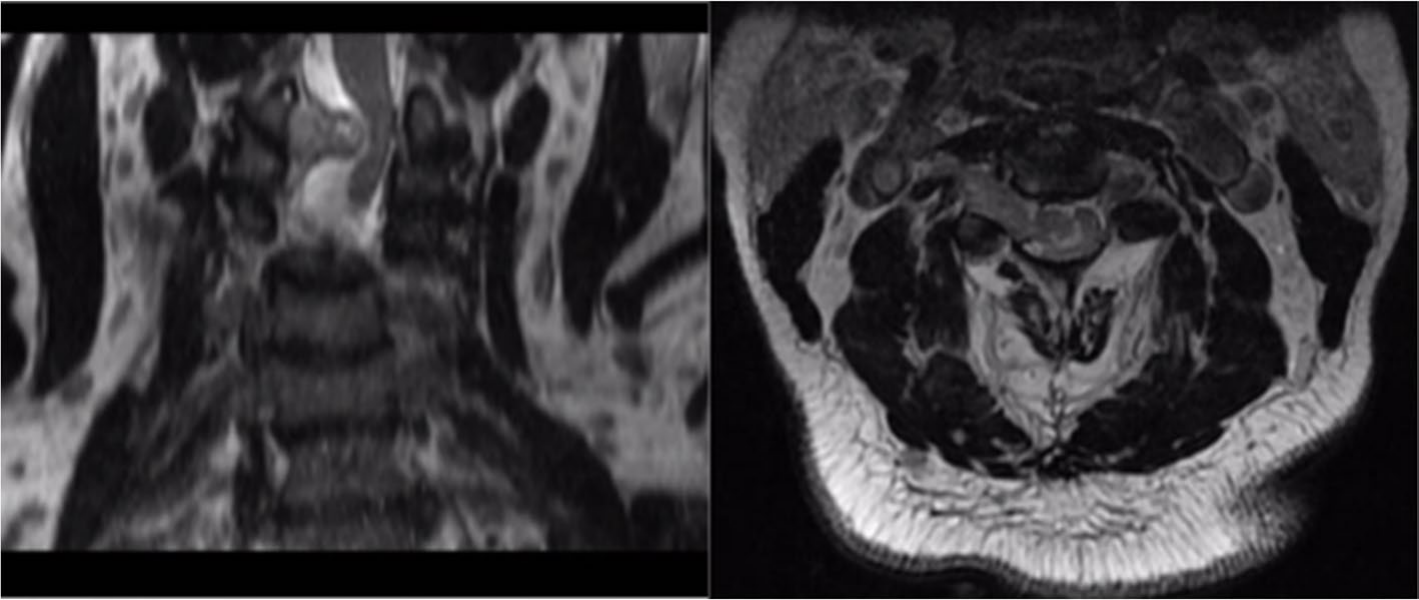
MRI showing (left and right T2 weighted cervical) spine was significant for an extradural 3-cm enhancing mass on the right at C2-C3 with severe compression of the cervical spinal cord and displacement of the cord into the left side of the canal, near to the vertebral artery
